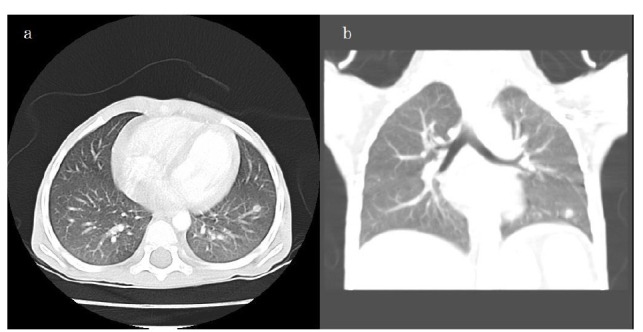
Spiral computed tomography scan of the chest and mediastinum without contrast (with coronal reconstruction)
